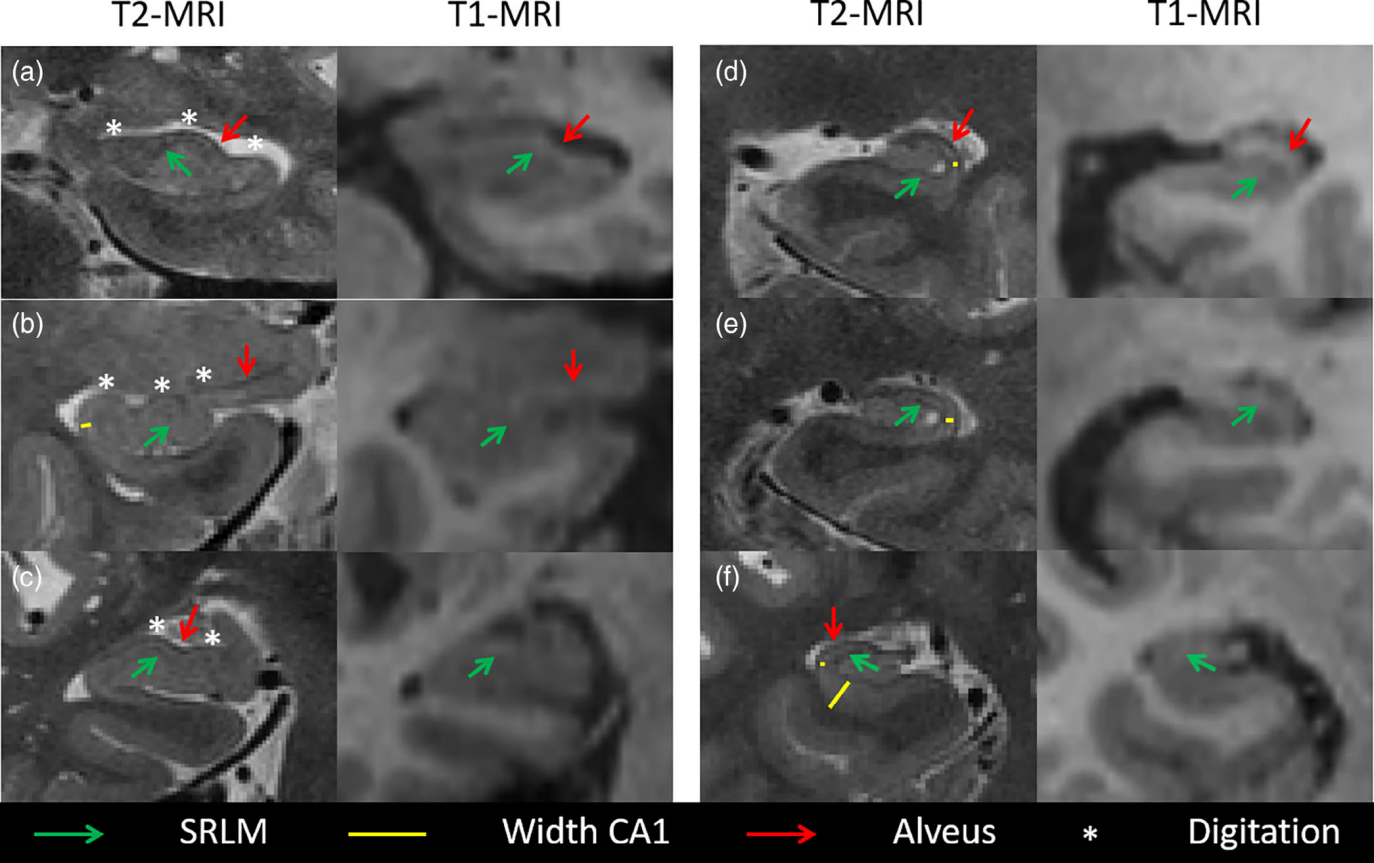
Comparison of 0.4 × 0.4 × 2.6 mm^3^ T_2_‐ and 1 × 1 × 1 mm^3^ T_1_‐weighted MRI in the same subjects demonstrating better visualization of the inner structure of the hippocampus on 0.4 × 0.4 × 2.6 mm^3^ T_2_‐weighted MRI; a and d‐f are cognitively normal (CN) older adults and b‐c are patients with Mild Cognitive Impairment (MCI). The green arrows point out the hypointense band stratum radiatum lacunosum moleculare (SRLM) which can be observed on the high‐resolution T_2_‐weighted MRI scans but not on the 1 mm^3^ isotropic T_1_‐weighted MRI scans. The visualization of SRLM allows for accurate depiction of the width of cornu ammonis (CA) as indicated by the yellow lines in T_2_‐weighted MRI but not on T_1_‐weighted MRI. Similarly, the high resolution and the depiction of SRLM and alveus (outer hypointense band, red arrows) in the T_2_‐weighted MRI, but not T_1_‐weighted MRI, allow for clear visualization of the digitations (white asterisks) in the hippocampal head, which is crucial for accurate segmentation. Note that the alveus is visible as a hyperintense band on T_1_‐weighted MRI but is less sharp than on the T_2_‐weighted MRI
